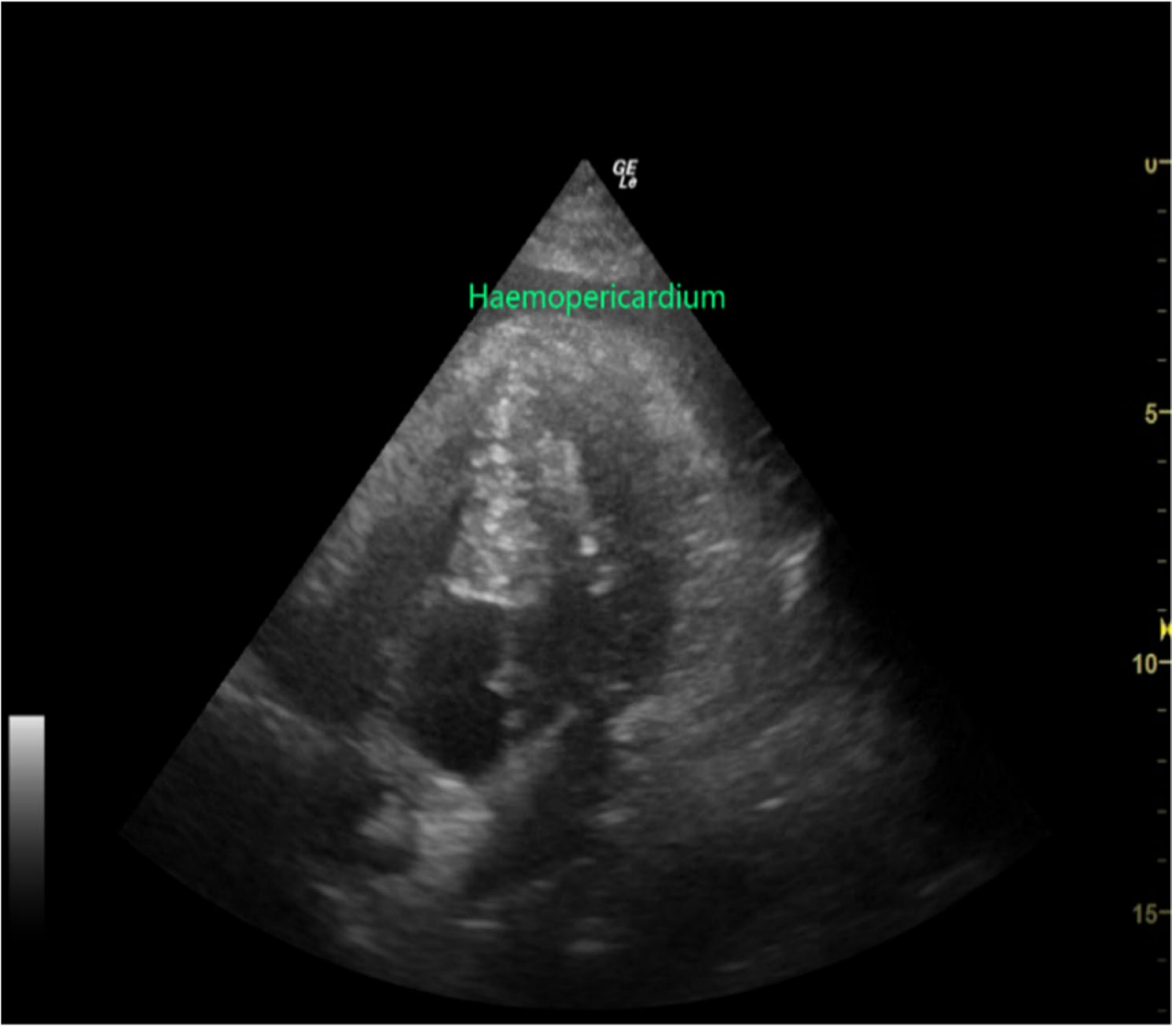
The intraoperative echocardiography revealed significant pericardial effusion
